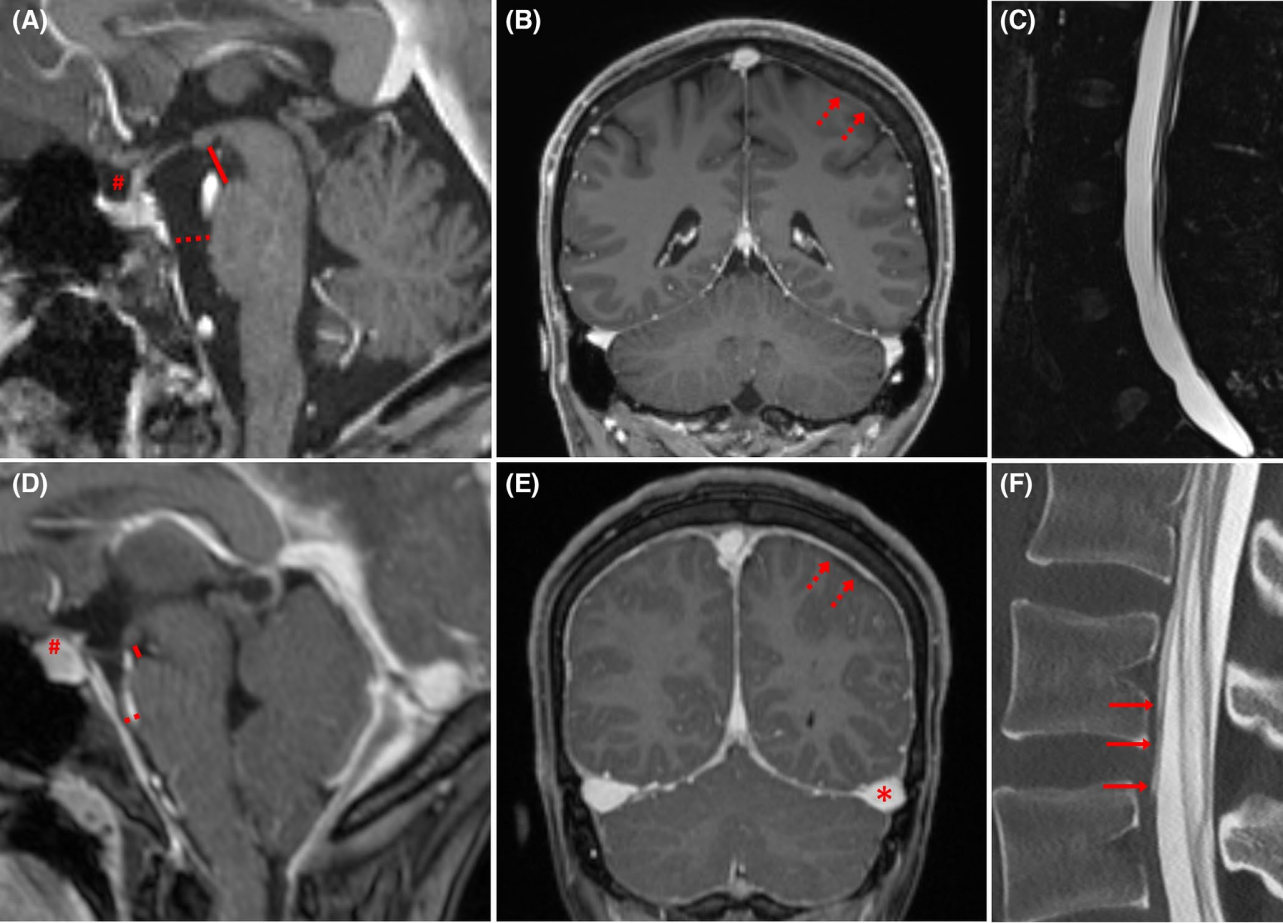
Imaging in two patients with chronic post‐dural puncture headache after unintentional dural puncture show representative examples of both, unremarkable (A‐C) and positive (D‐E) findings for spinal CSF loss. Contrast‐enhanced sagittal (A) and coronal (B) T1‐weighted MPRAGE in a 31‐year‐old woman shows normal measurements of the suprasellar (rhomb), prepontine (red dotted line), and mamillopontine (red line) distances in (A) as well as unenhanced dura (dotted arrow in B). Sagittal 3D T2‐weighted SPACE fs at a spatial resolution of 0.63 mm is negative for epidural lumbar fluid (C). Contrast‐enhanced sagittal (D) and coronal (E) T1‐weighted MPRAGE in a 27‐year‐old woman discloses positive findings of the head for a spinal CSF leak including diminished mamillopontine, prepontine, and suprasellar distances as well as engorgement of the venous sinus (asterisk) and pachymeningeal enhancement (dotted arrow) with small subdural hematoma/hygroma (D). Moreover, contrast egress is visible in the sagittal CT myelography after intrathecal injection of iodine contrast medium in the ventral epidural space at the level L1/2 (arrows in F). CSF, cerebrospinal fluid; CT, computed tomography; fs, fat‐sat; MPRAGE, 3D Magnetization Prepared Rapid Gradient Echo; SPACE, 3D Single slab turbo spin echo (TSE) sequence with a slab selective, variable excitation pulse. [Color figure can be viewed at wileyonlinelibrary.com]
